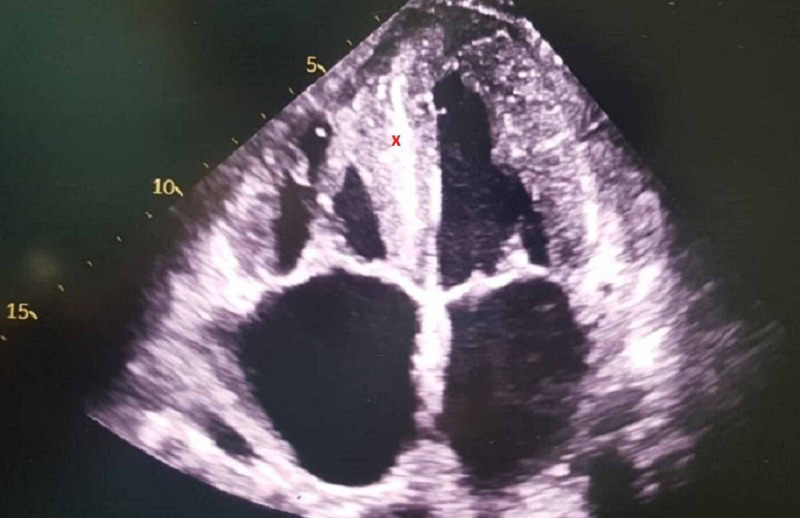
Four chamber view echocardiography showing septal hypertrophy x: Septal hypertrophy
